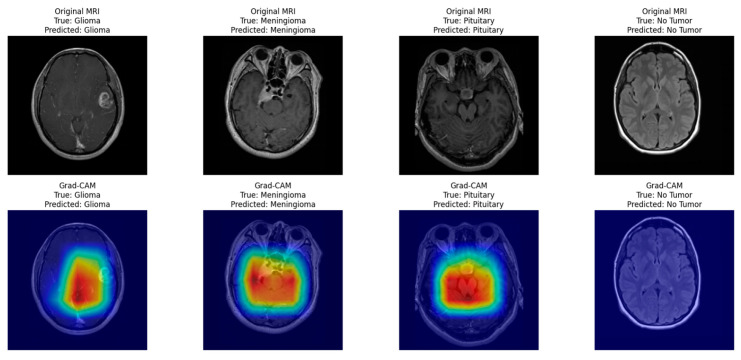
Grad-CAM visualizations for representative MRI test samples generated using the EfficientNet-B1 classification model.
